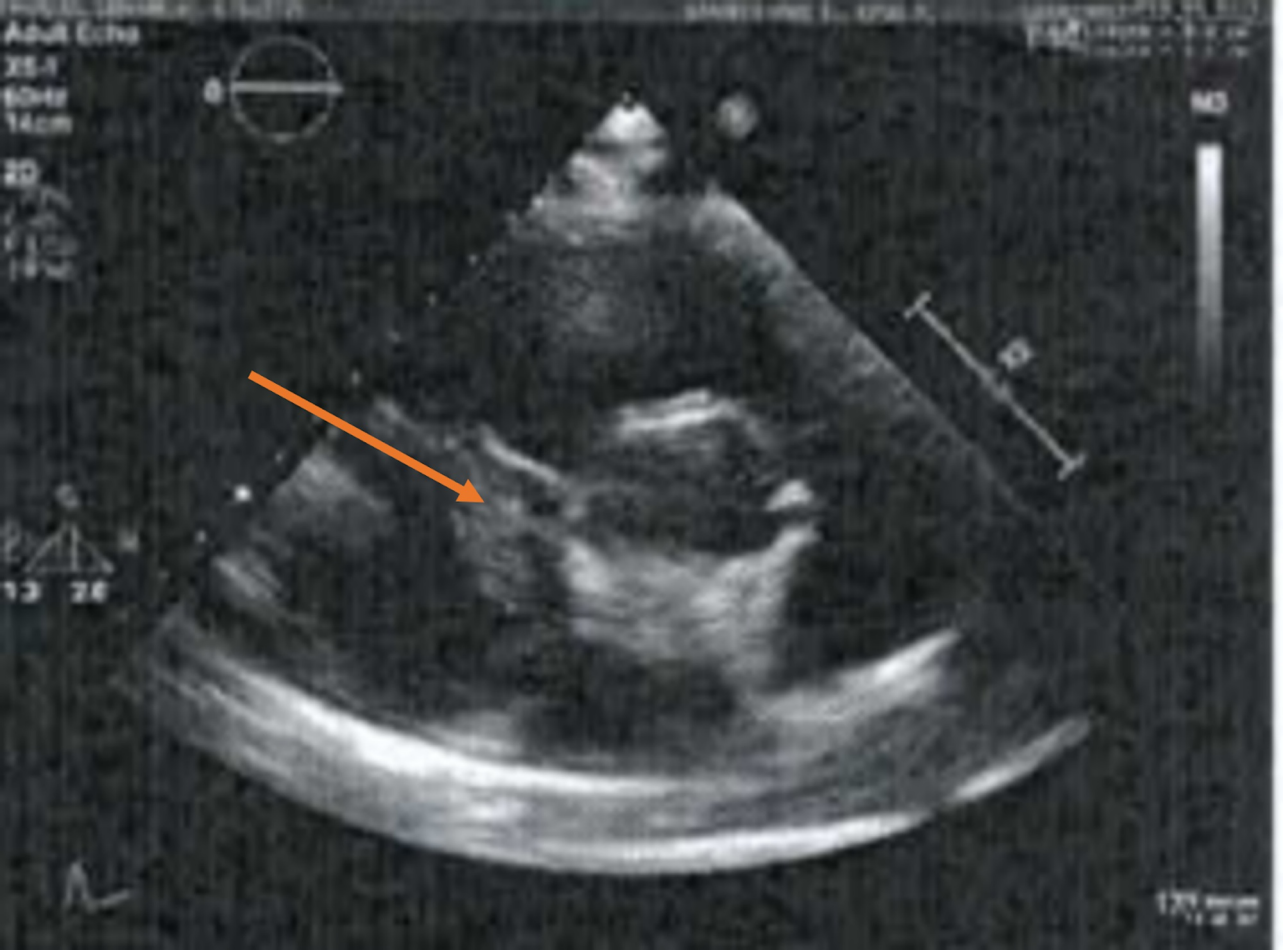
POCUS in the emergency department showing tricuspid valve vegetation POCUS- Point of care ultrasound
